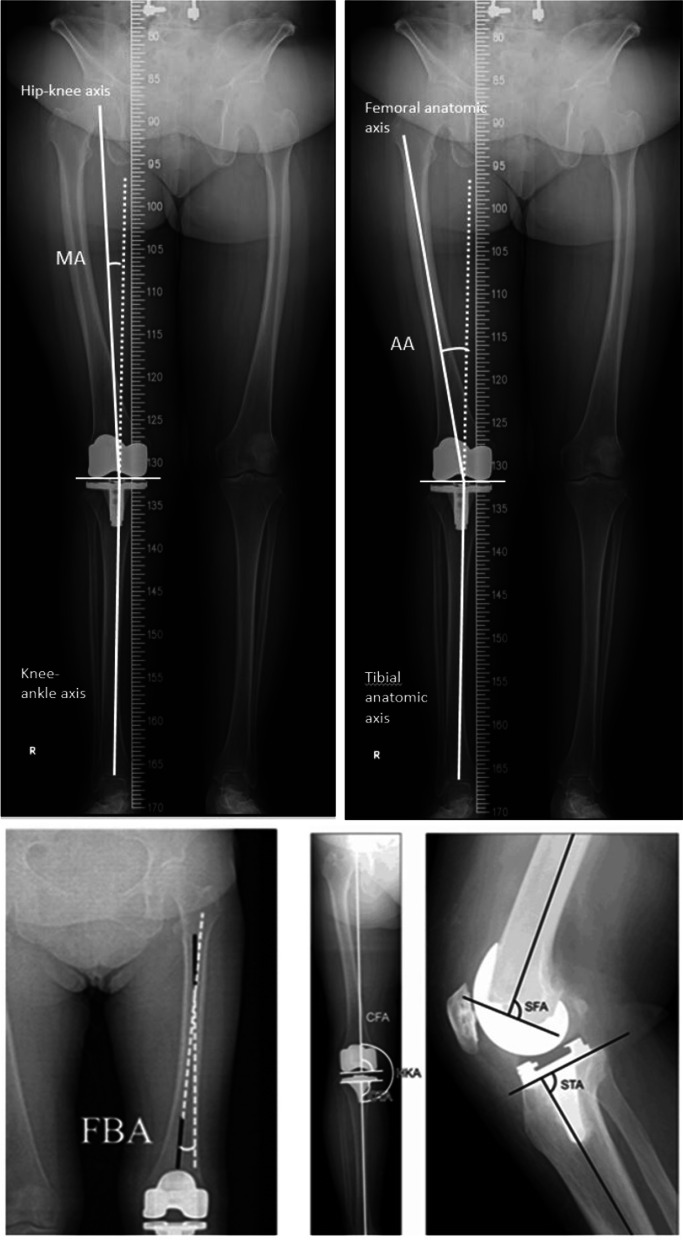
The radiographic parameters of total knee arthroplasty. MA: mechanical alignment (the angle between hip-knee axis and knee-ankle axis on the scenography), AA: anatomical alignment (the ankle between femoral axis and tibial axis on the scanography,), FBA: femoral bowing angle (the ankle between the axis of proximal third femur and the axis distal third femur), CFA: coronal femoral component angle (the angle of femoral component formed with the anatomical axis of femur on coronal view), CTA: coronal tibia-component angle (the angle of femoral component formed with the anatomical axis of tibia), SFA: sagittal femoral component angle (the angle between anatomical axis of the femur and perpendicular line to femoral prosthesis on the lateral view), STA sagittal tibial component angle (the angle between anatomical axis of the femur and perpendicular line to tibial prosthesis)
